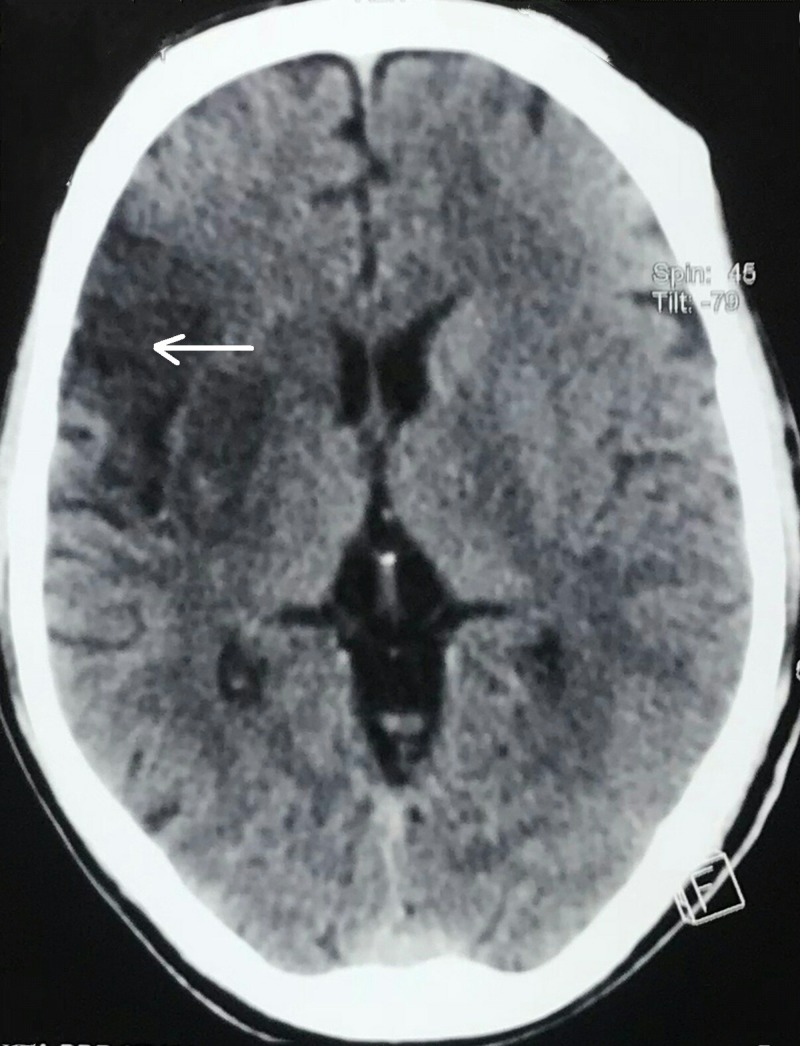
A computed tomography (CT) scan of the head showed right-sided ischemic stroke (white arrow) involving more than one-third of the middle cerebral artery territory
